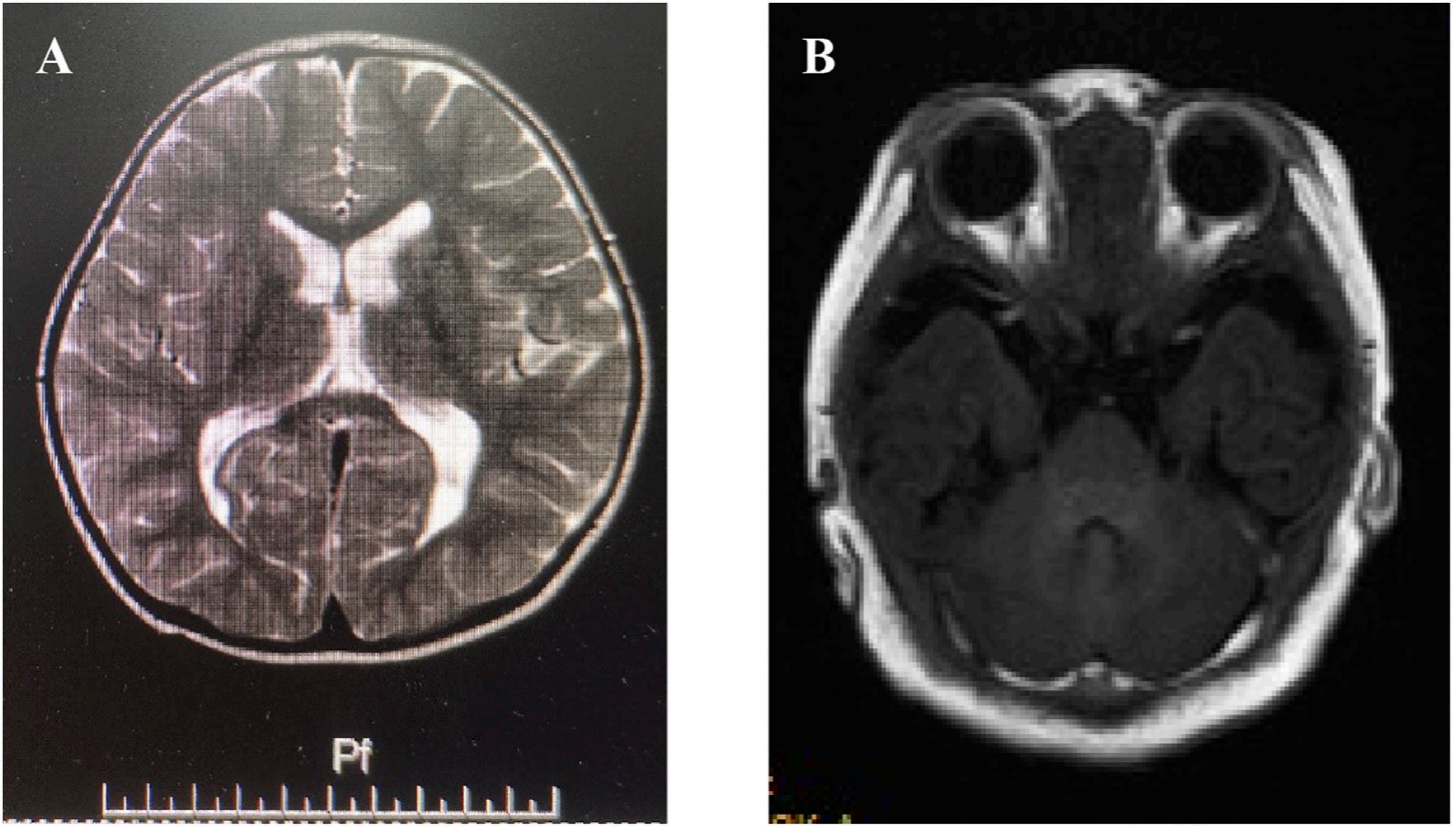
Imaging features of proband II & III. (A) Brain magnetic resonance imaging (MRI) displayed small focal signal abnormalities in the bilateral occipital lobes in proband II. (B) Brain MRI showed slightly thinner genu and splenium of the corpus callosum along with irregular bilateral lateral ventricles, mildly enlarged posterior horn, and widened left temporal subarachnoid space in proband III.
